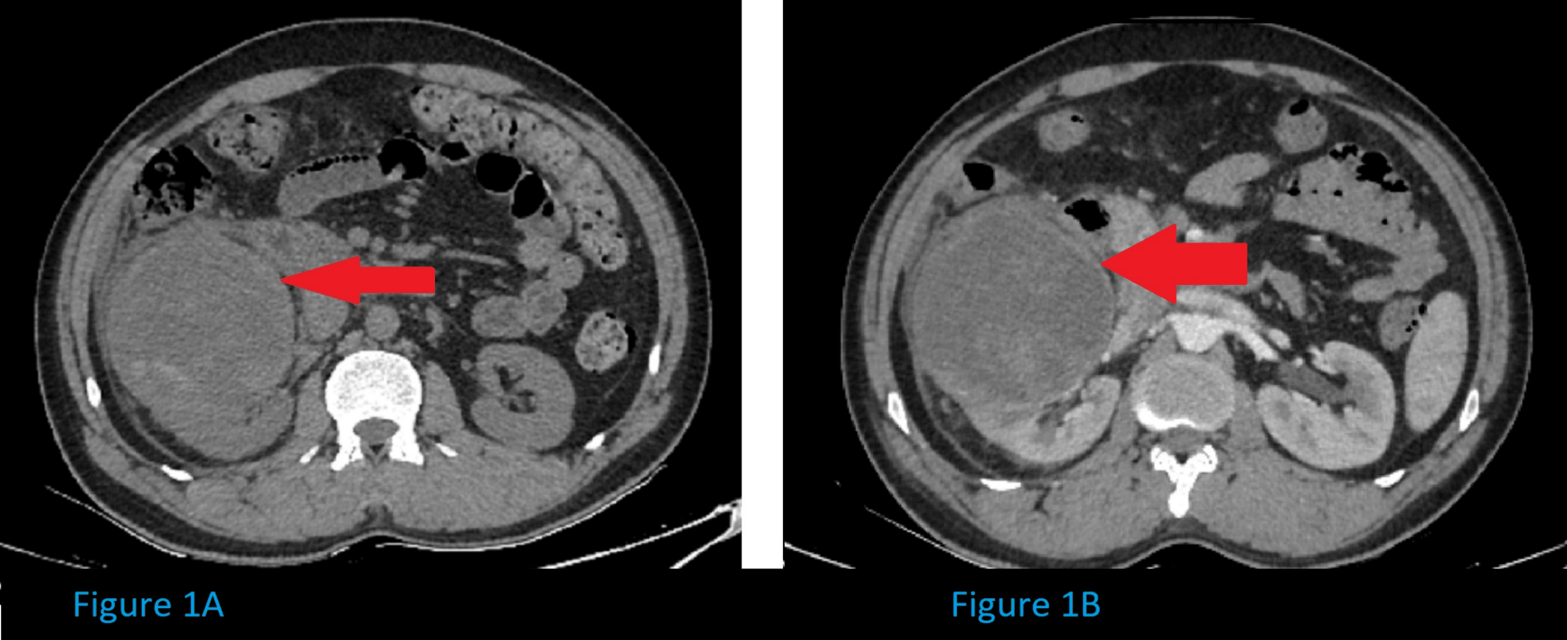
Non-contrast CT scan images (1A) Shows a large heterogenous lesion arising from the inter pole region with few hyperdense solid components and septations within. (1B) The contrast-enhanced computed tomography (CECT) image shows no significant enhancement of the lesion.
